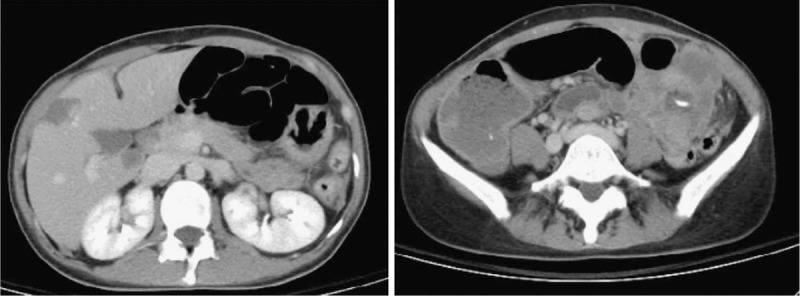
CT scan revealed 7 cm mass lesion of the sigmoid colon with visceral peritoneum and suspected pericolic/perirectal, superior rectal lymph node lesions. There was low grade bowel obstruction with fluid retention in the distal bowel loops. Bulky lymph nodes were noted over retroperitoneum, bilateral iliac chains, and right obturator region. Distant metastases were also suspected in hypodensity hepatic and splenic lesions.
